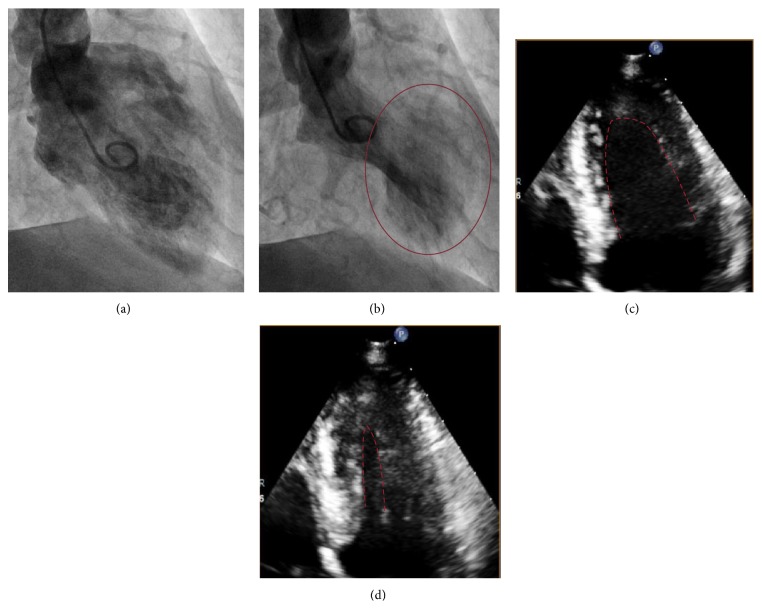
Left ventricular catheterization shows (a) the end of diastole and (b) apical ballooning at the end of systole. Transthoracic echocardiogram shows (c) LV end of diastole and (d) LV end of systole (no apical ballooning).
